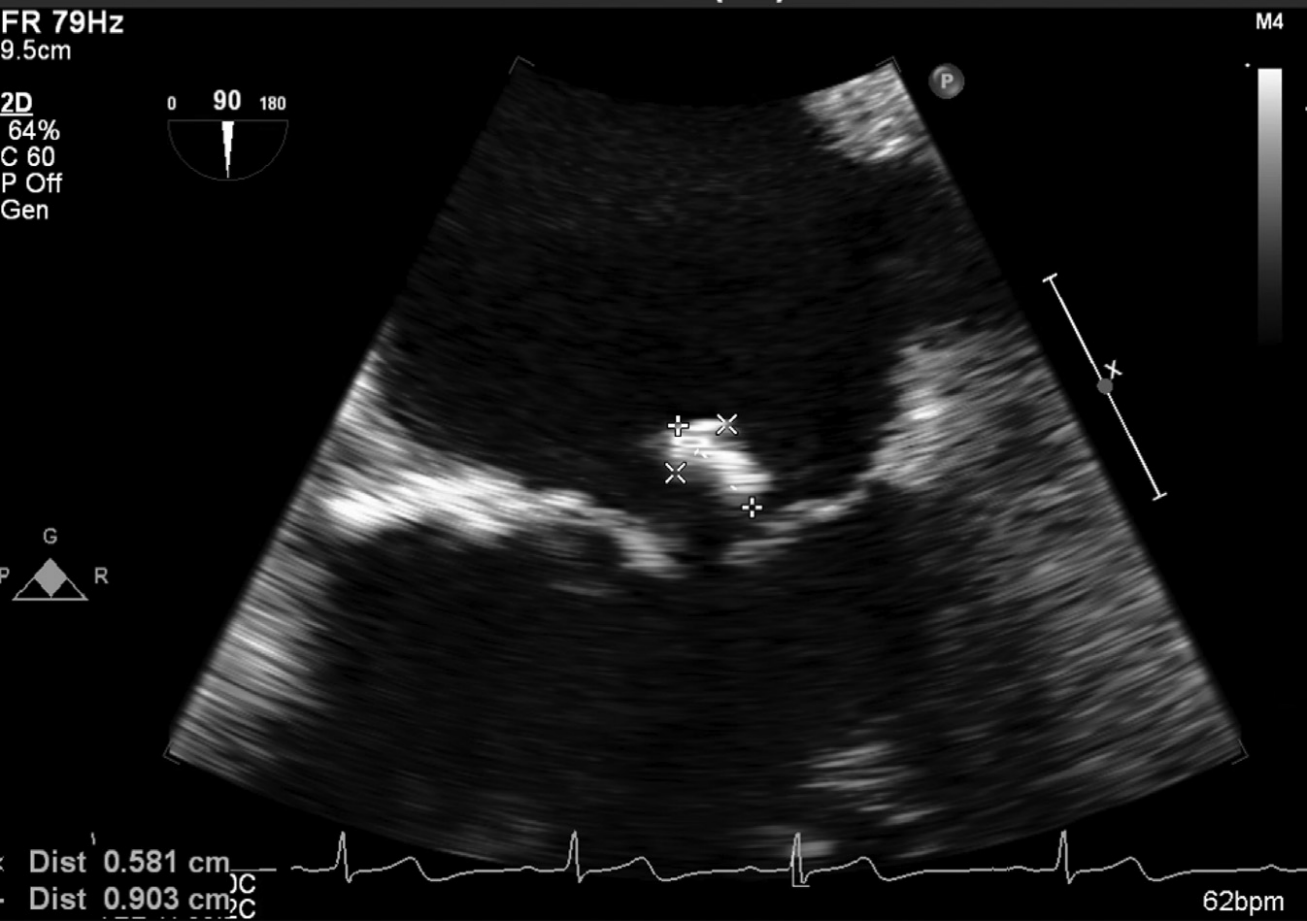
Preoperative image demonstrating a mitral valve mass. Transesophageal echocardiogram image at 90° showing size of mass attached to the posterior leaflet prior to WATCHMAN (Boston Scientific, Marlborough, MA) insertion.
